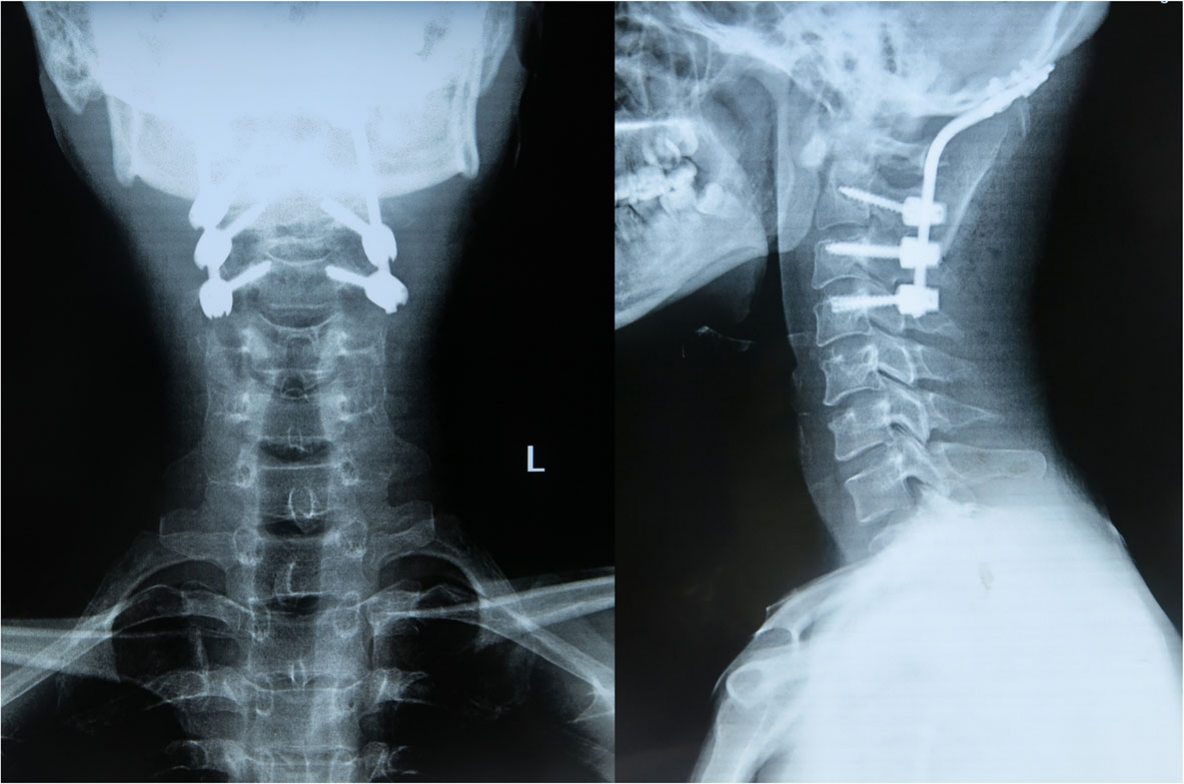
X-ray examination one week after operation showed good recovery of cervical physiological curvature, good internal fixation position, and satisfactory stability reconstruction of the upper cervical spine
